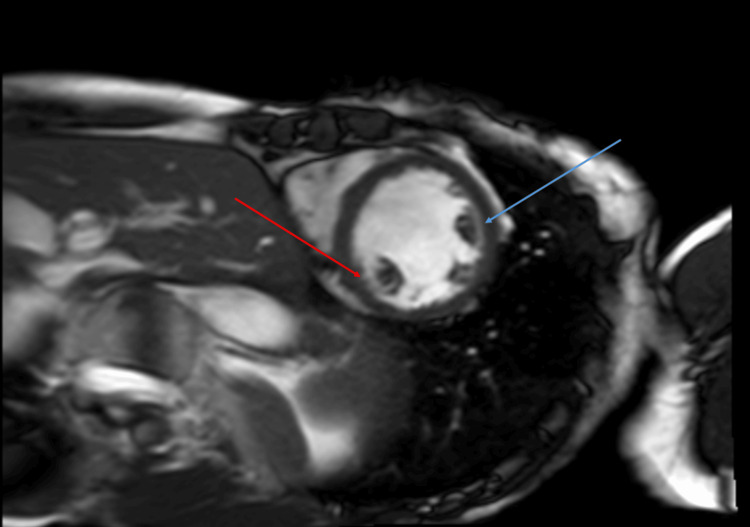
Cardiac MRI short axis: Phase-sensitive inversion recovery (PSIR) Red arrow: Subendocardial late gadolinium enhancement involving the mid-inferoseptal wall segment. Blue arrow: Mid-myocardial late gadolinium enhancement involving the mid-anterolateral wall segment.
